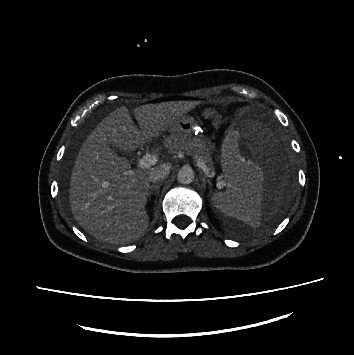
CT abdomen (axial) demonstrating subcapsular hematoma.
